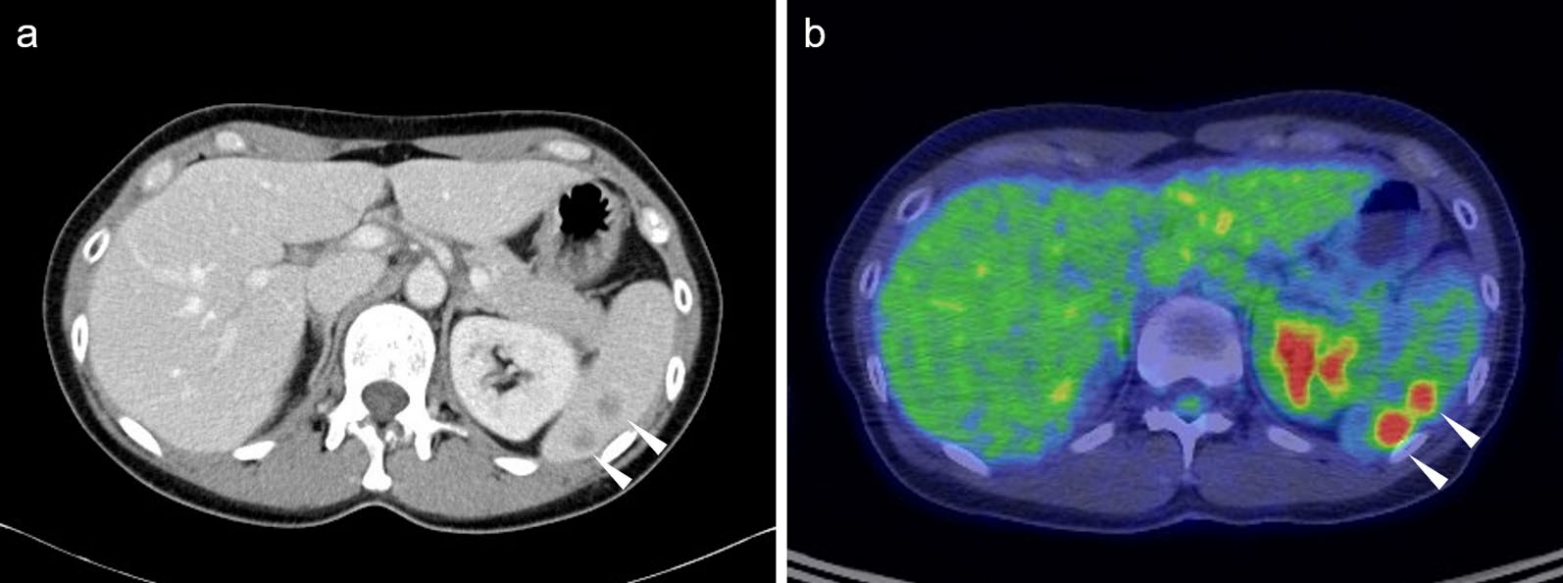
CT and PET/CT findings of case 2. a CT image shows small low-density lesions. b PET/CT shows high 18F-fluorodeoxyglucose uptake at the site of the mass (maximum standardized uptake value: 7.07–8.51) in case 2. Arrowheads indicate splenic tumors. *CT* computed tomography, *PET/CT* positron emission tomography/computed tomography
